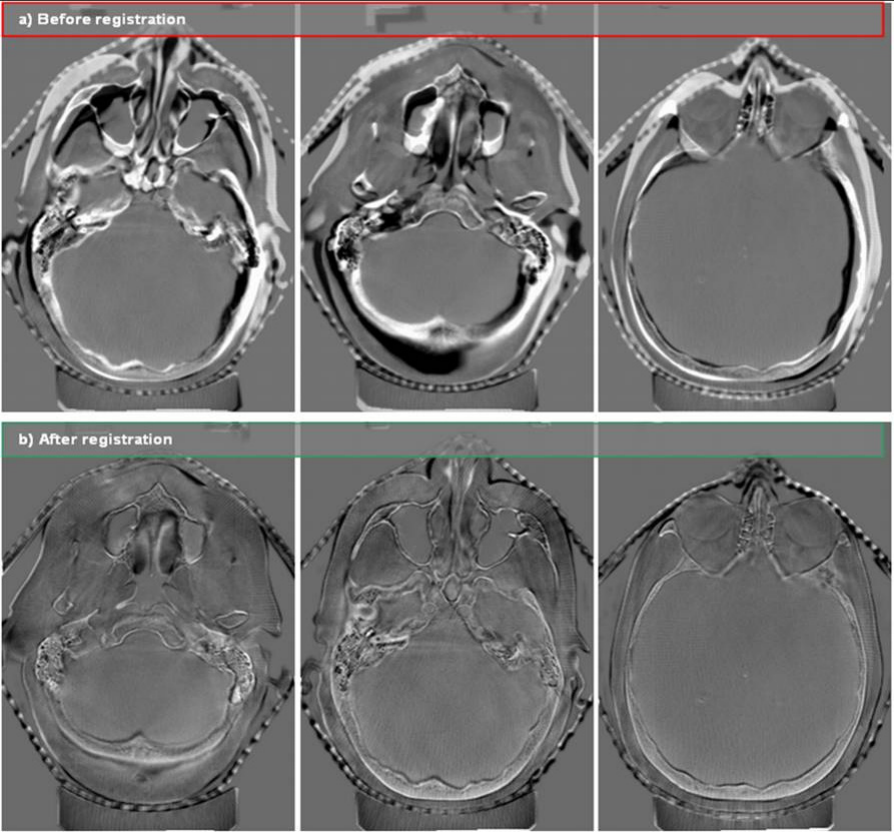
Subtraction images of representative case‐study cone beam computed tomography and planning computed tomography slices (a) before and (b) after registration.
